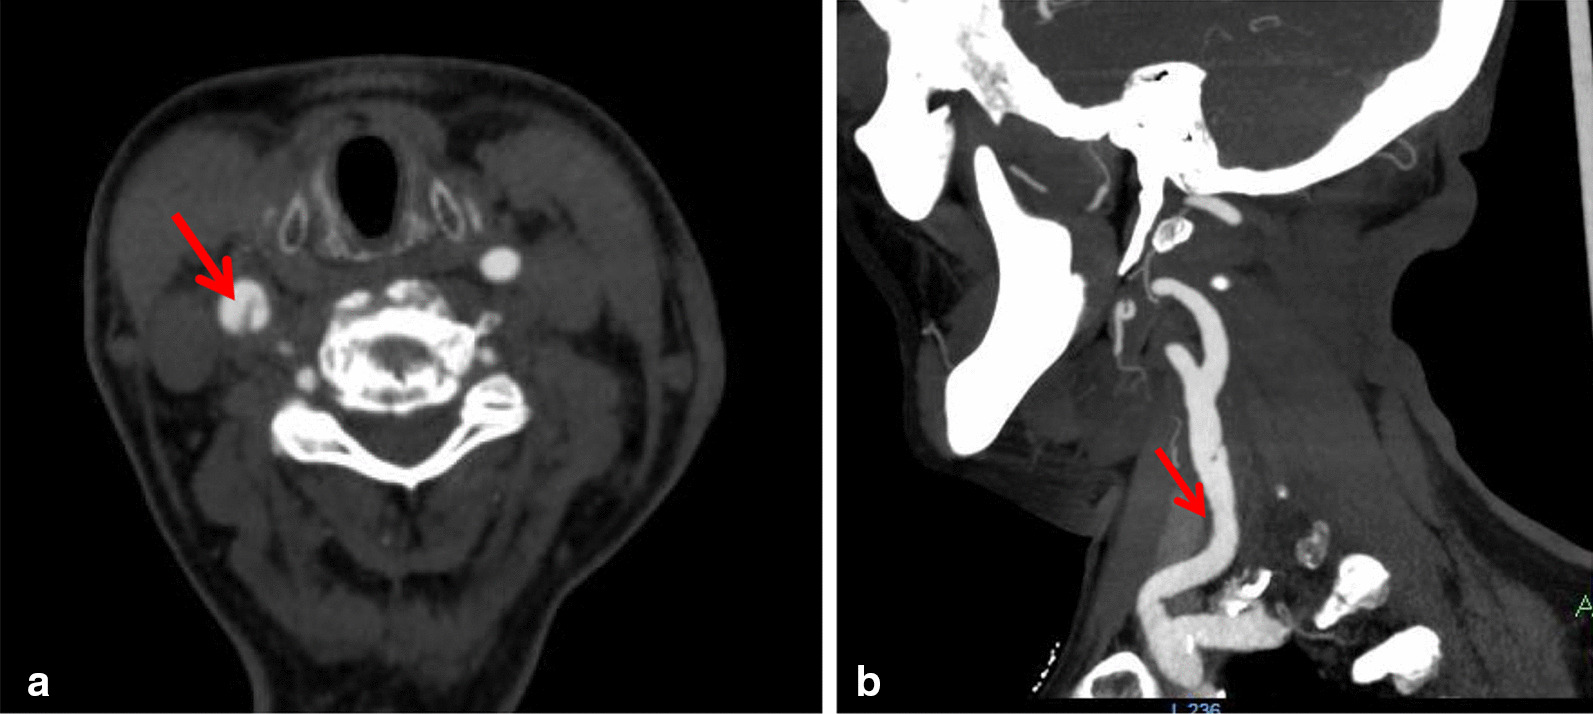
a CTA axial scan shows the filling defect of a thin-film contrast agent in the right carotid artery lumen, the main body of which is located deep into the lumen. b Sagittal CTA of the same patient showed that the web-like structure was located in the lumen at the end of the common carotid artery
